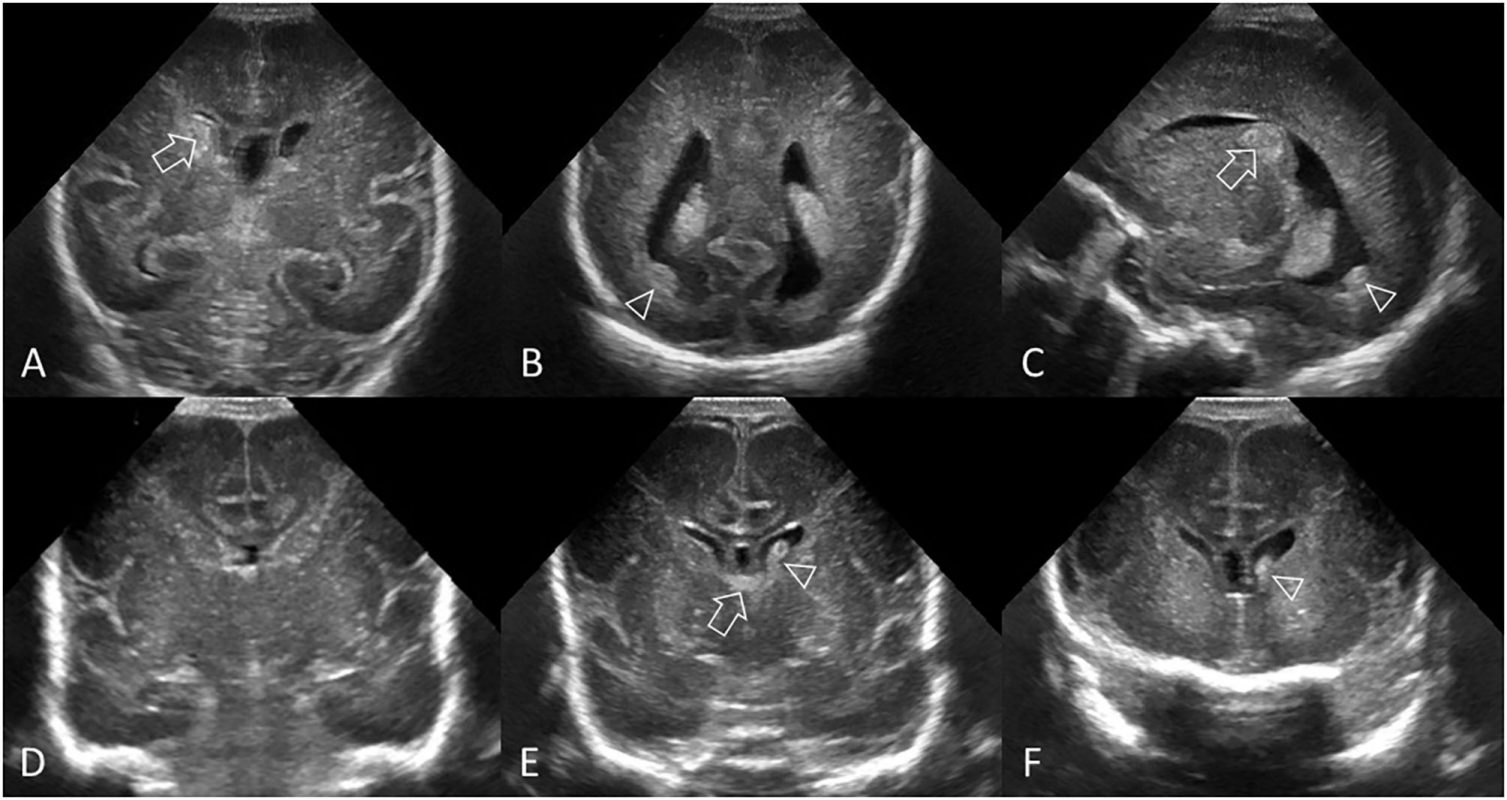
Grade II GMH-IVH. (A–C) Ultrasound scan in a preterm infant (GA 26 weeks) with common pattern of grade II GMH-IVH. (A) Coronal scan at the level of the frontal horns of the lateral ventricles shows echogenic clot involving the caudate nucleus (arrow). No ventricular dilatation. (B) Coronal scan at the level of the trigone of the lateral ventricles shows a small volume of intraventricular clot layering dependently (arrowhead). (C) Parasagittal scan through the right lateral ventricle shows the same clot centered at the caudothalamic notch (arrow) and the intraventricular hemorrhage within the occipital horn separate to choroid plexus (arrowhead). (D–F) Ultrasound scan in a different preterm infant (GA 27 weeks) with delayed pattern of Grade II GMH-IVH. (D) Coronal scan, at the level of the frontal horns of the lateral ventricles, was initially normal 2 days after birth. No GMH-IVH was detected. (E) Follow-up coronal scan 6 days after birth shows echogenic clot in the left lateral ventricle (arrowhead). The AHW is <6 mm (measurement not shown). Normal choroid plexus in the roof of the third ventricle (arrow). (F) Additional coronal view of the frontal horns anterior to the foramen of Monro shows further echogenic intraventricular clot (arrowhead). There is no extension of choroid plexus anteriorly past the caudothalamic grooves (i.e., the frontal and occipital horns have no choroid plexus), thus echogenic material in these sites suggests IVH. This infant had two cUS exams in the first week after birth. The cUS scan showing the greatest severity of GMH-IVH in the first week (i.e., cUS at 6 days after birth in this case) should be considered for GMH-IVH classification purposes.
